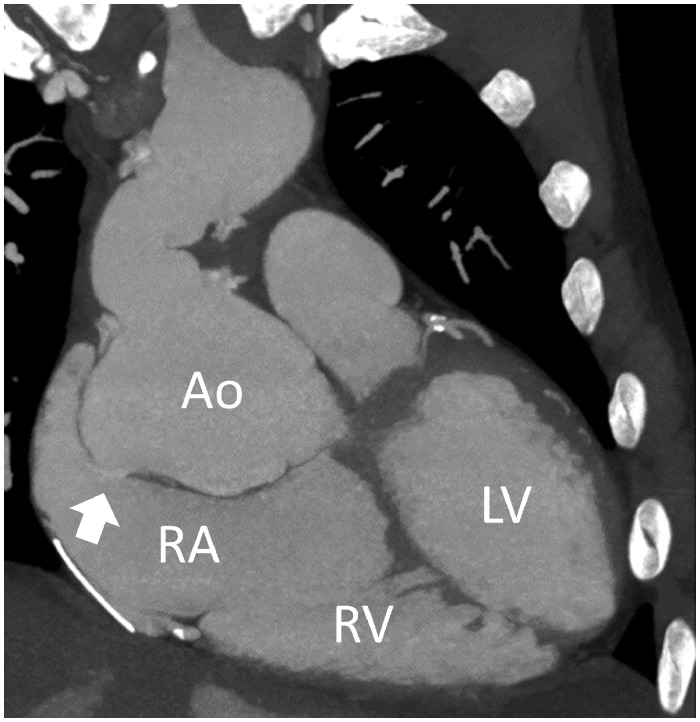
The coronal reconstruction of cardiac computed tomography showed dilated Valsalva sinus of non-coronary cusp which ruptured to the right atrium (white arrow). Ao, aorta; LV, left ventricle; RA, right atrium; RV, right ventricle.
